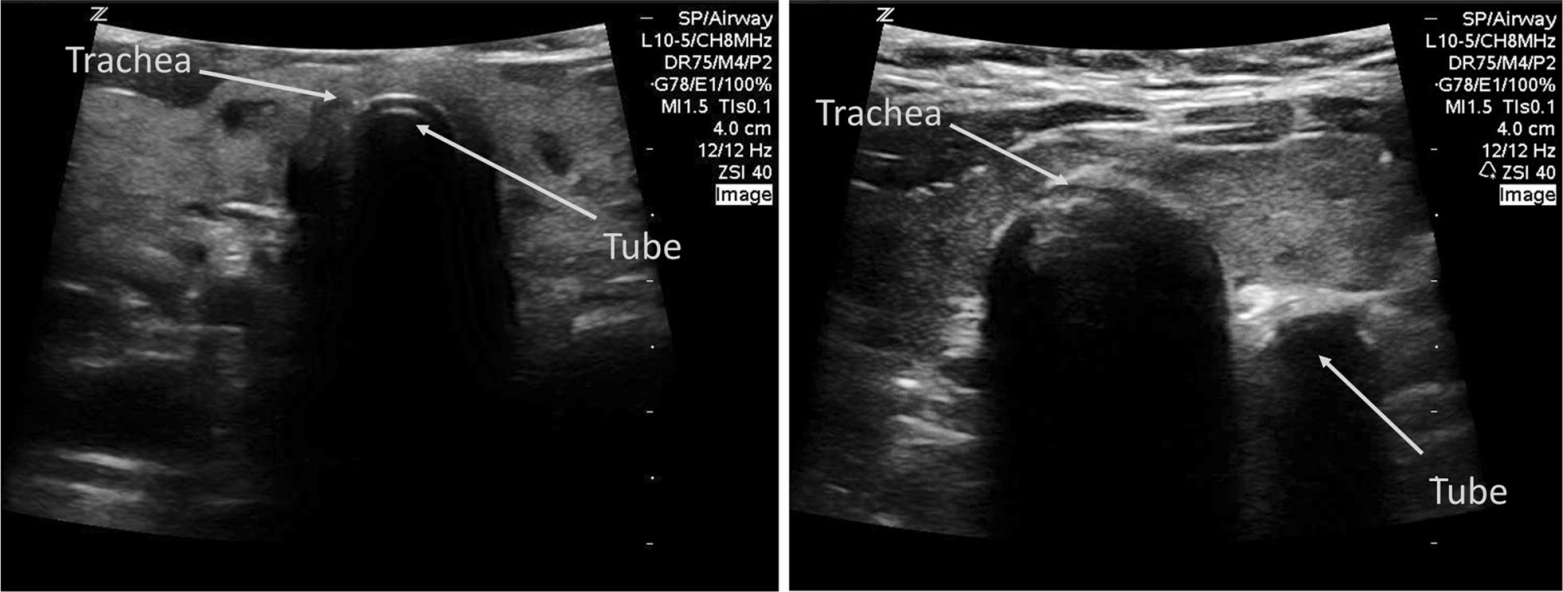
Ultrasound images of endotracheal and esophageal intubations. Images are generated by placing a high-frequency linear transducer in the transverse position (indicator facing the patient’s *right side*) at the level of the suprasternal notch. On the *left image*, a normal endotracheal intubation is shown with the echogenic semicircular tube visible within the lumen of the trachea. On the *right image*, an esophageal intubation can be identified by the presence of a ‘double tract sign’ with a second semi-circular acoustic shadow appearing outside of the trachea
